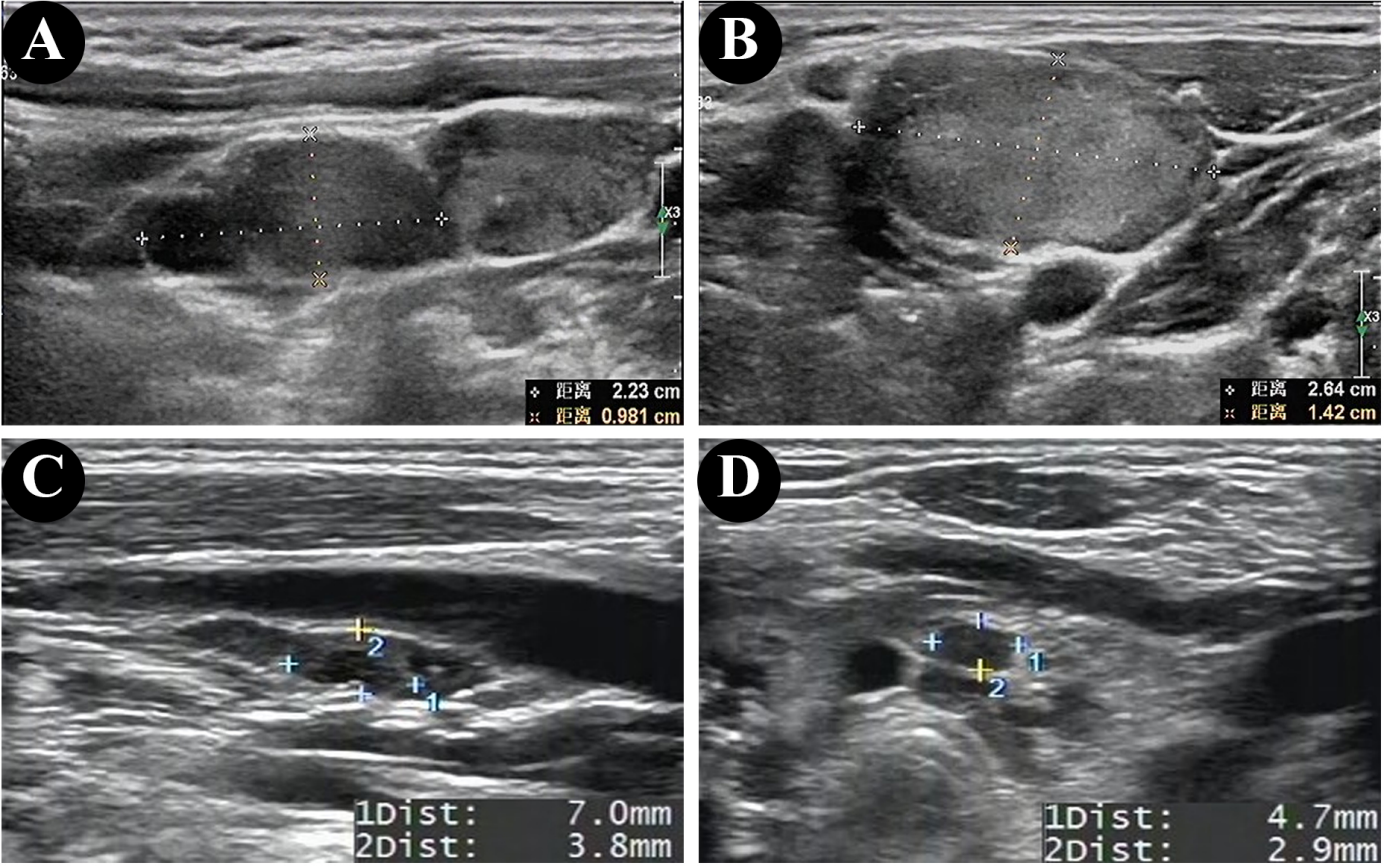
Ultrasonographic findings of lateral lymph node metastasis in patients without HT (A, B) and central lymph node metastasis in patients with HT (C, D).
